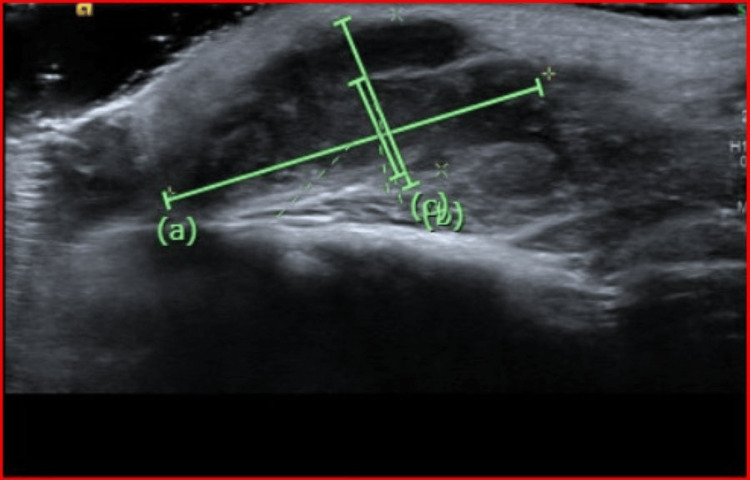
Ultrasound of the right posterior auricular region. Ultrasound of the right posterior auricular region showing abscess with measured dimensions of (a) 2.97 cm, (b) 1.36 cm, and (c) 0.78 cm within the right retroauricular lymphadenitis with extension into the adjacent musculature.
